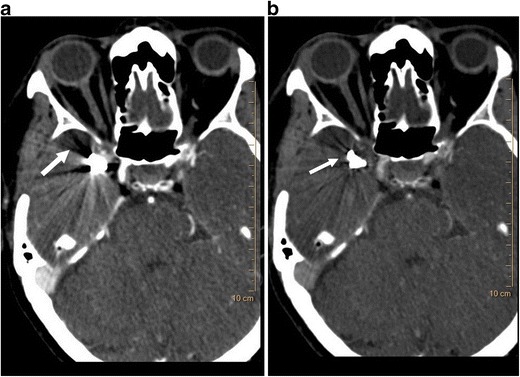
Metallic artefact reduction. (a) Axial 120-kVp routine diagnostic CT image through the head shows dense metallic opacity in the right temporal region (*arrow*). (b) 120-keV VMI at the same level shows significant improvement in the metallic artefact (*arrow*)
